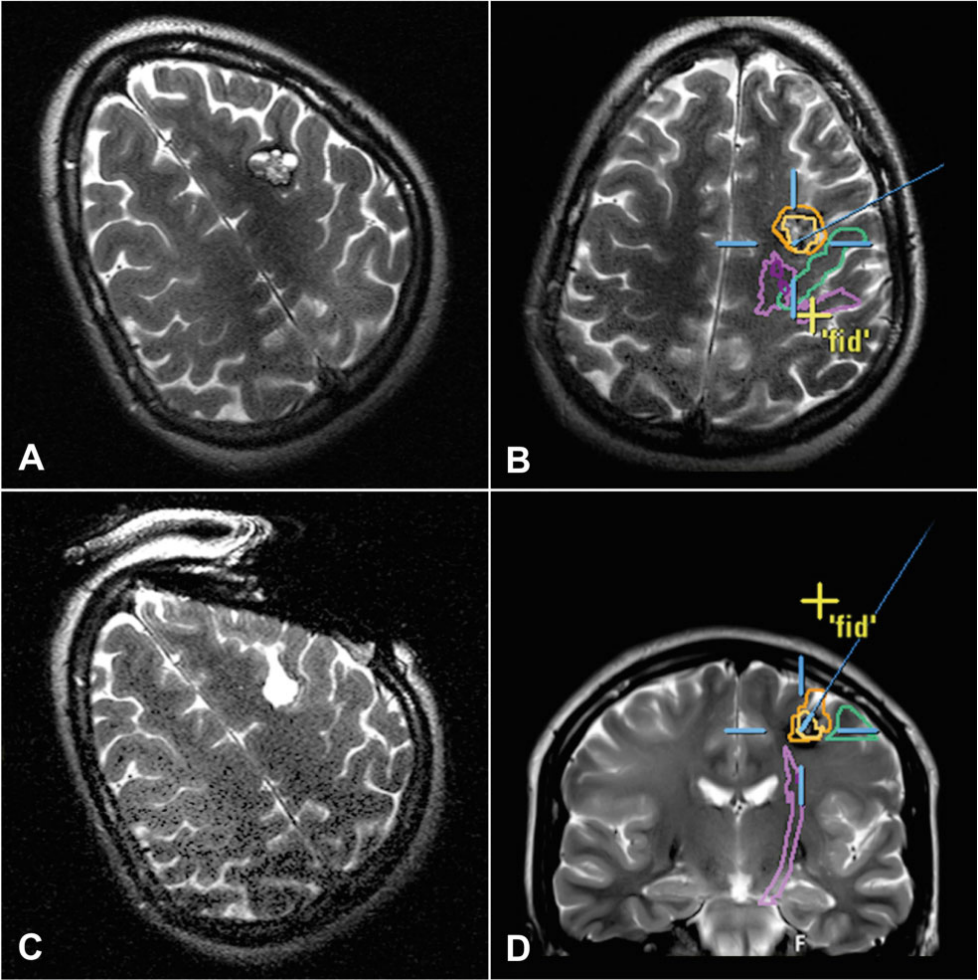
Axial (A–C) and coronal (D) T2-weighted intraoperative 1·5T MRI sequences of patient No. 18 with a precentral cavernous hemangioma. Prior to beginning of surgery (B, D), the cavernoma (orange) with the surrounding hemosiderin rim (ocher) has been segmented manually and implemented into the neuronavigation plan along with functional magnetic resonance imaging and diffusion tensor imaging data of the primary motor area (green) and the pyramidal tract (purple). The ideal trajectory and the target point (cross) is marked in light blue. Images A and C document complete extended lesionectomy [see online for colour version].
